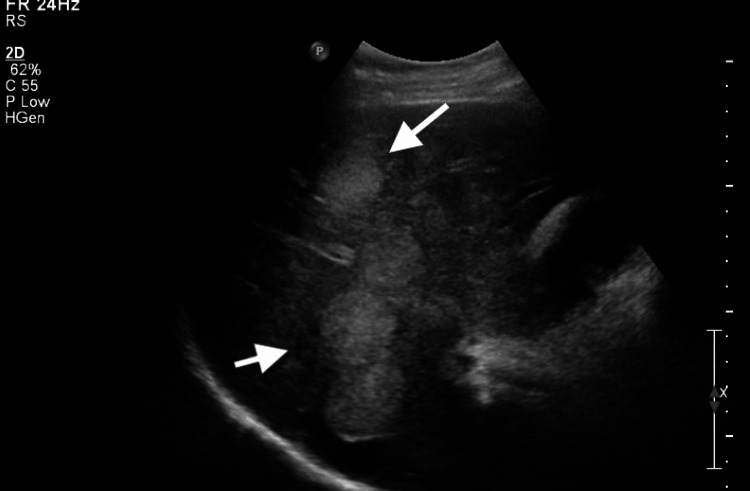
Ultrasound of the liver demonstrating multiple hypoechoic masses in the left hepatic lobe (white arrows).
